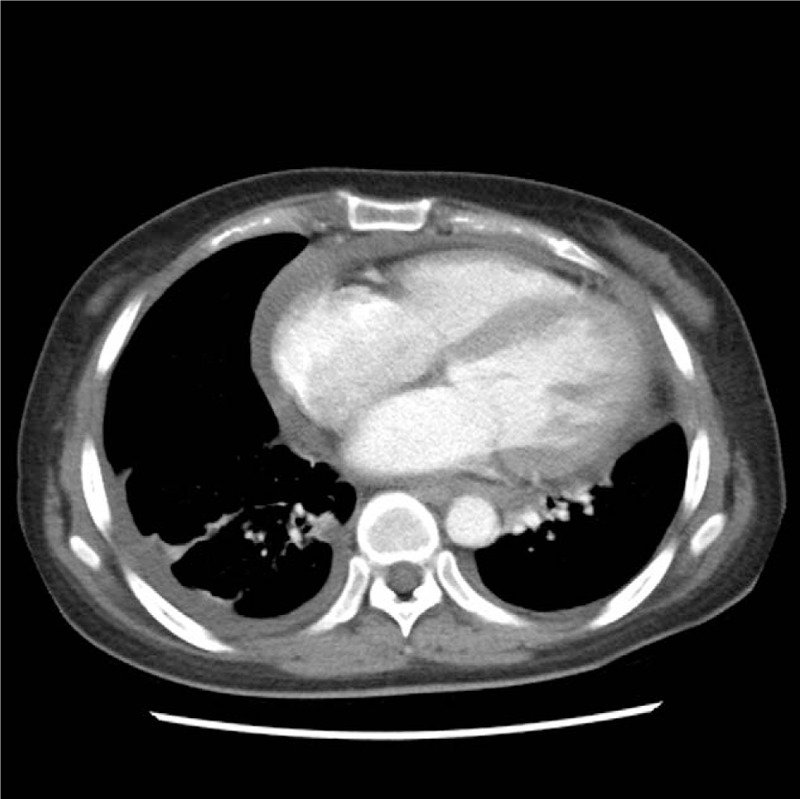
Chest computed tomography findings. Cardiomegaly with pericardial effusion, bilateral pleural effusions, probably loculate, without discernible pleural thickening.
